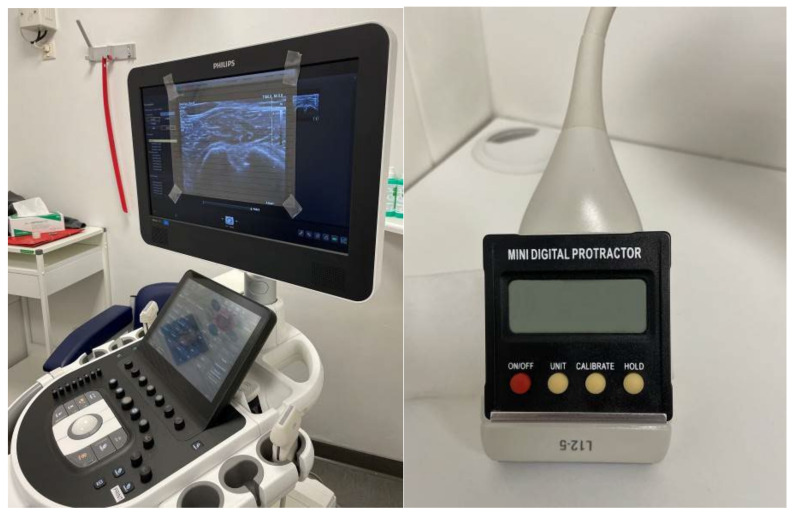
Equipment for sonographically assisted measurement of humeral torsion.
